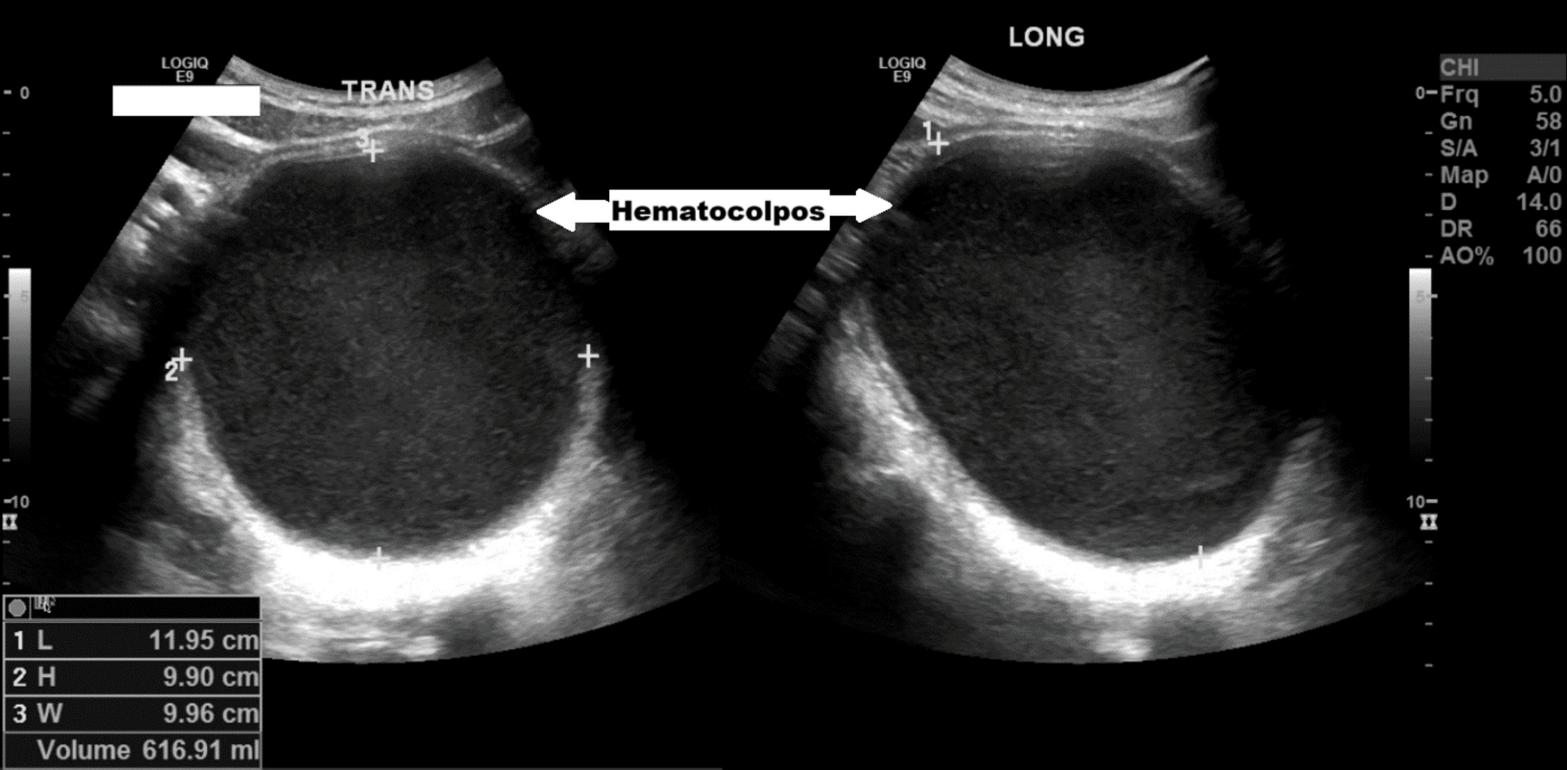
Pelvic ultrasound showing a well-defined cystic lesion with internal echoes, measuring 9.90 × 9.96 × 11.95 cm (estimated volume approximately 617 mL), arising from the cervical region, consistent with hematocolpos.
